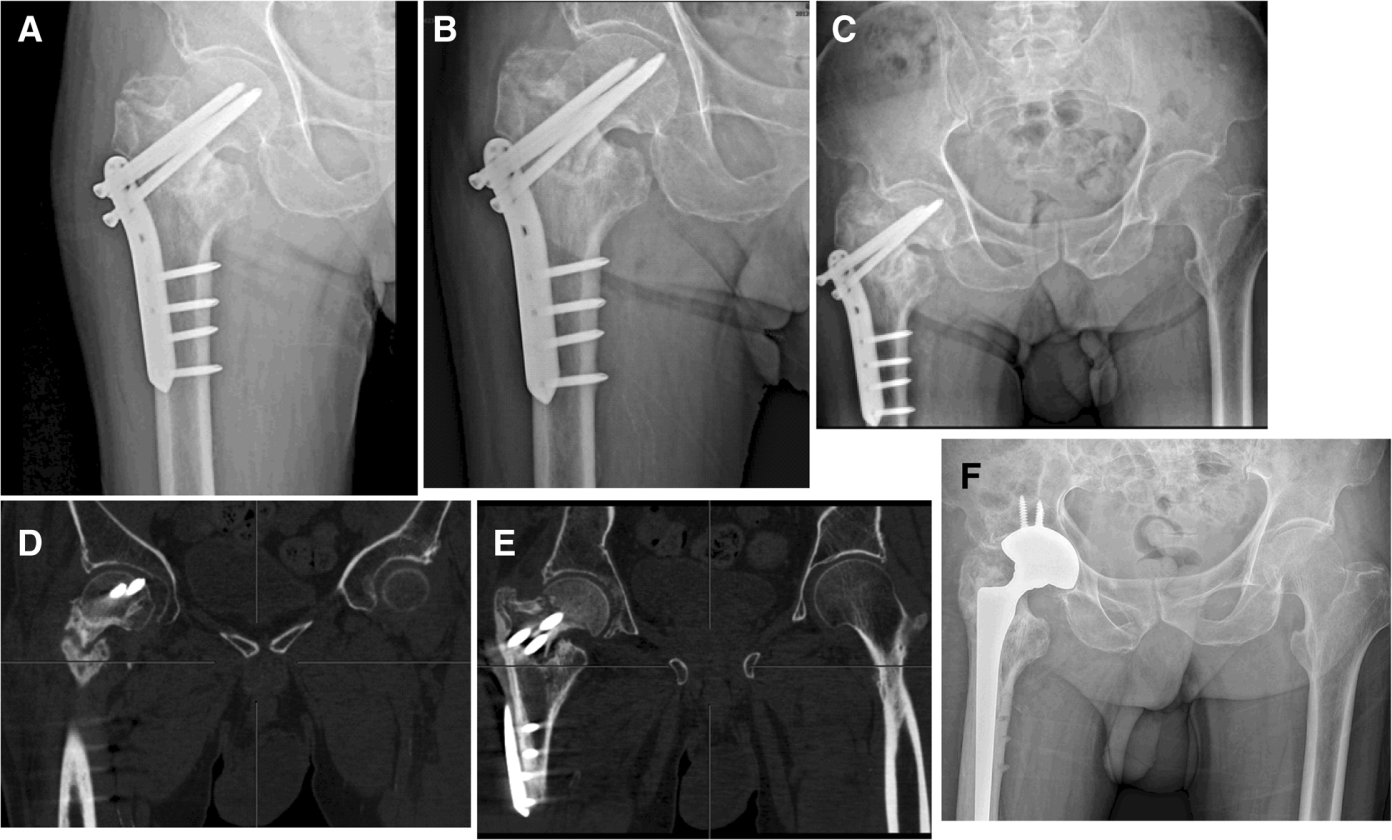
A 79-year-old male with AO/OTA type 31A2.3 suffered mechanical failure and nonunion. a Postoperative radiograph showing loosening of proximal screws and varus collapse 36 weeks after surgery. b, c Postoperative radiograph showing that progressive loosening and penetration through femoral head of proximal femoral screws as well as shortening of femoral neck (44 and 56 weeks after surgery, respectively). d, e CT scan showing nonunion and penetration through femoral head (56 weeks after surgery). f Revision surgery of THA
